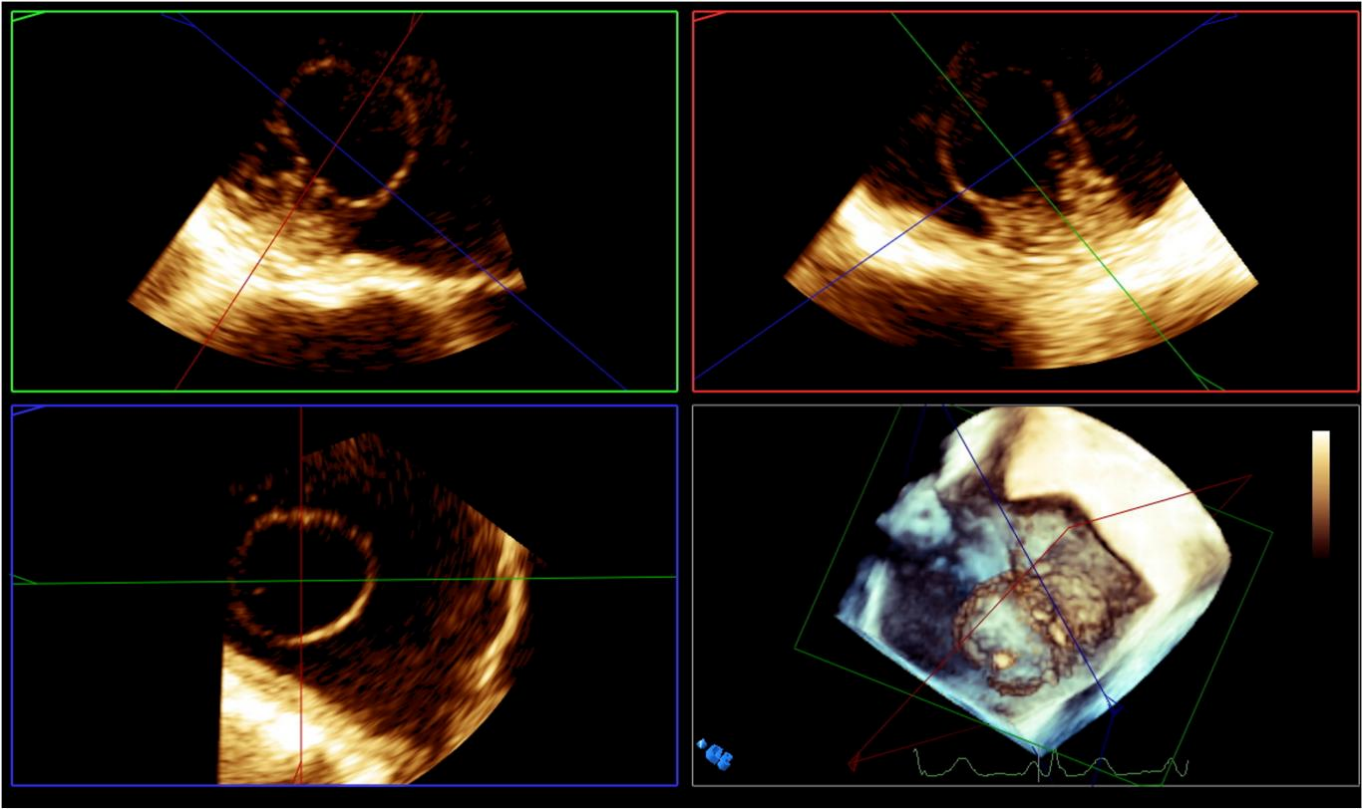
A three-dimensional image of this tumour by transoesophageal echocardiography showed the light bulb–like morphology.
